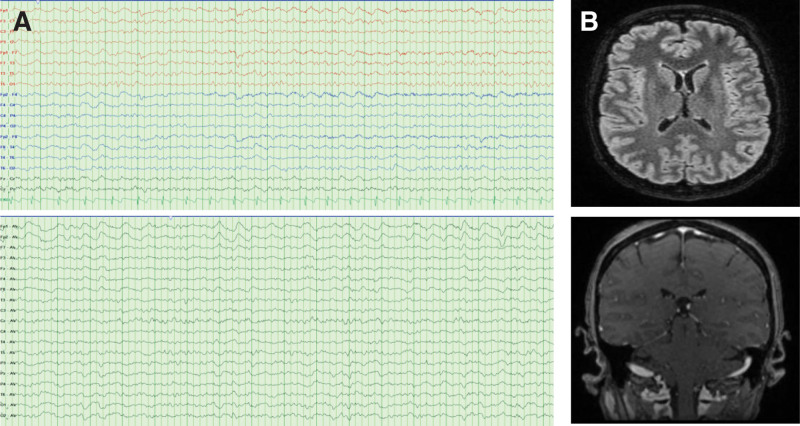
(A) Anterior-posterior bipolar montage and referential montage EEG with intermittent bursts of bilateral frontal intermittent rhythmic delta activity (FIRDA) over an underlying mild diffuse slowing of background activity; (B) FLAIR-T2 weighted axial view and T1-weighted dynamic contrast-enhanced coronal view brain MRI with normal results. EEG = electroencephalogram, MRI = magnetic resonance imaging.
